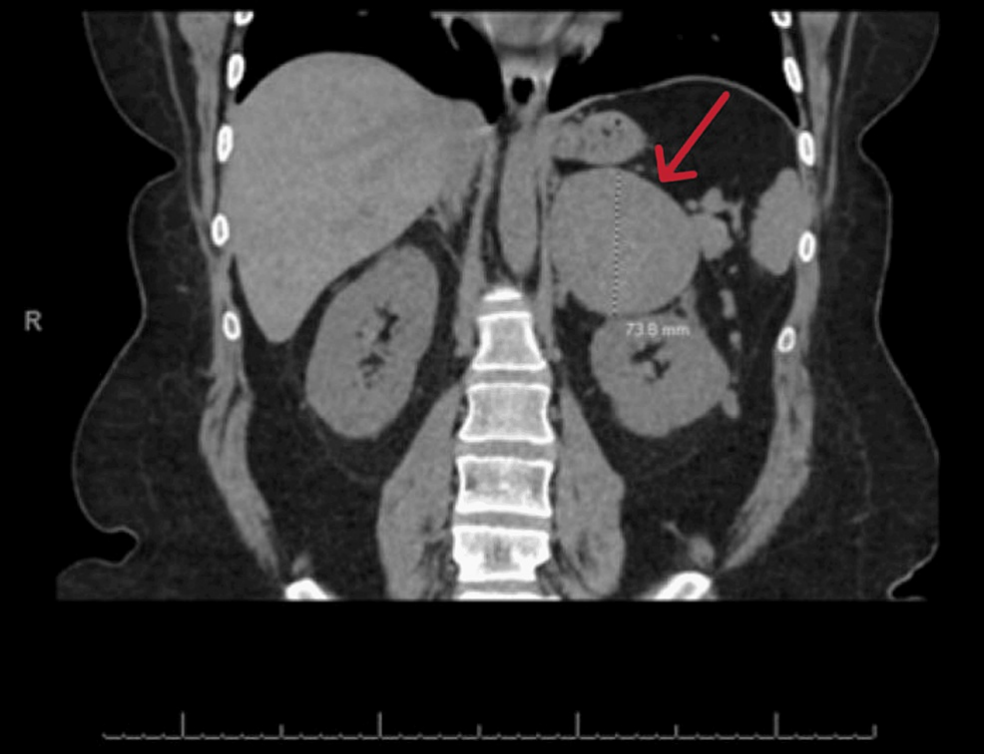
CT scan of the abdomen (coronal view) with contrast showing the left adrenocortical carcinoma (red arrow).
